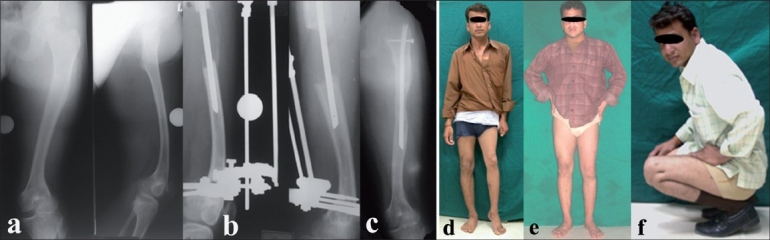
(a) Preoperative AP and lateral X-rays of left thigh including hip and knee. (b) Lengthening performed over a modified Humerus nail 7.5 cm length achieved. External fixator was removed in 13 weeks. (c) AP X-ray of left thigh showing bone consolidation with nail in situ. (d) 22 year old male with 8 cm shortening due to growth arrest following childhood septic arthritis of the lower femoral physis. (e,f) Final clinical result showing limb alignment and knee range of motion
